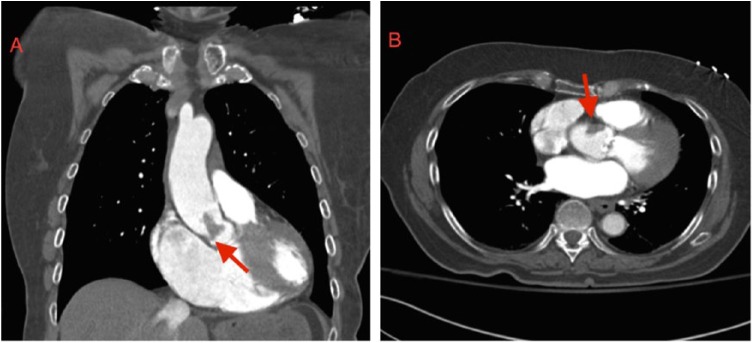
Contrast-enhanced computed tomography revealed the presence of an aortic root mass 21 × 16 mm suggestive of a thrombus, extending into the right coronary sinus of Valsalva (red arrow; A) with near complete obliteration of the left atrial appendage with another large thrombus (red arrow; B).
